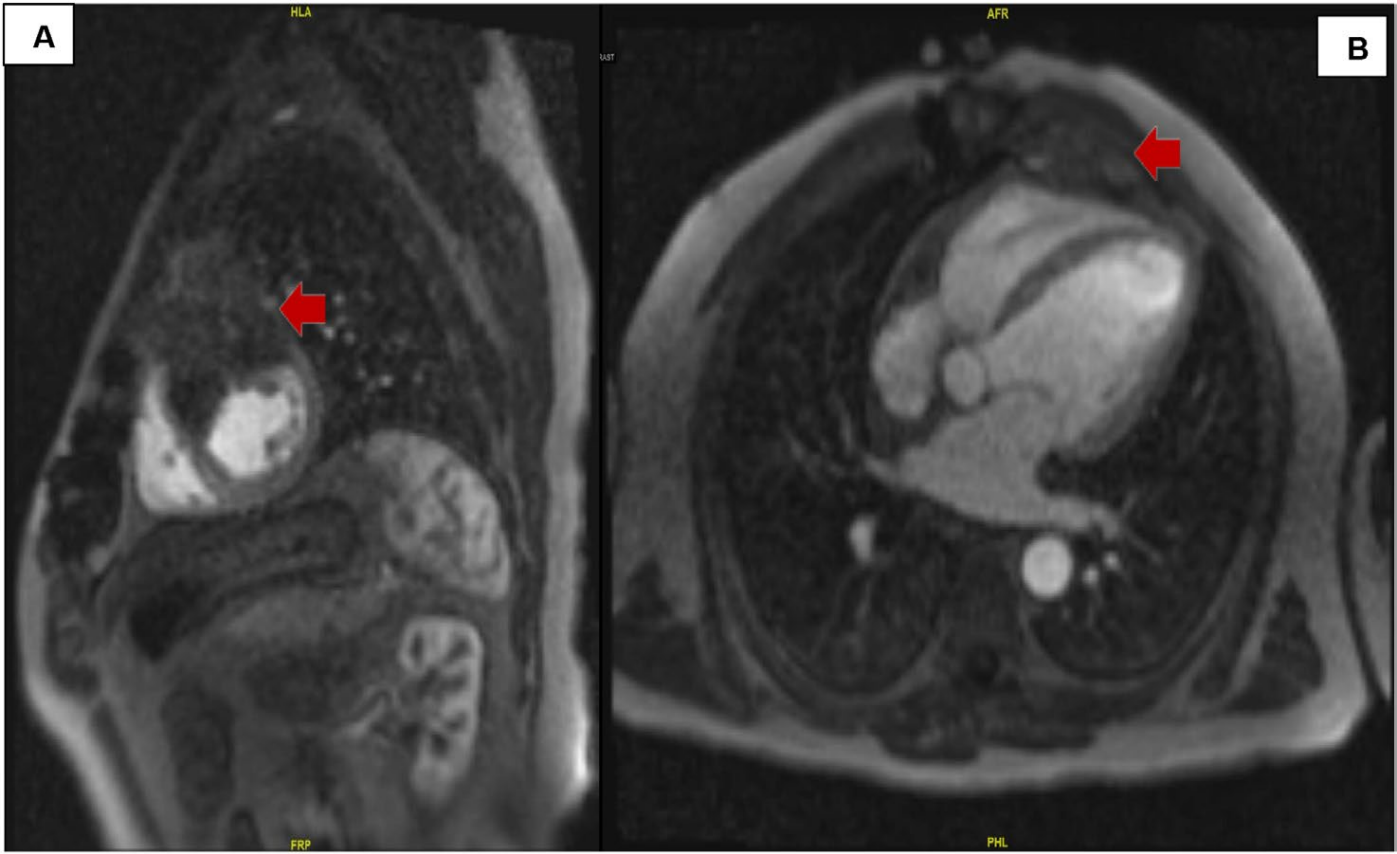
(A, B) Cardiac MRI revealing anterior mass invading the cardiac chambers (red arrow).
